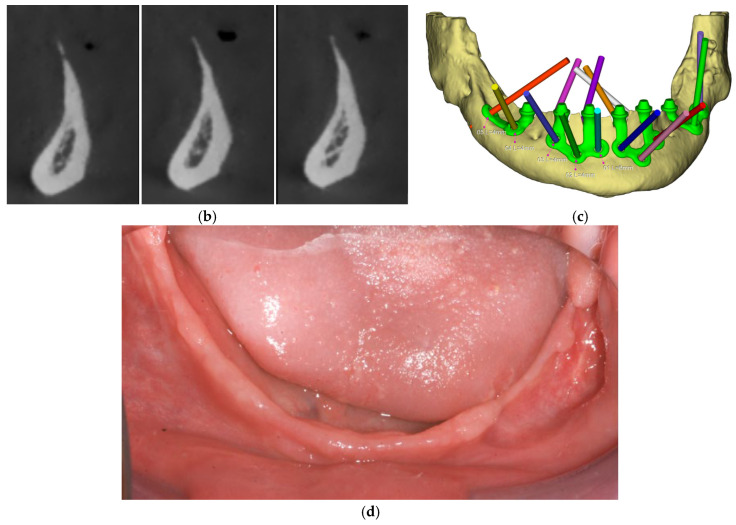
(a) A panoramic radiograph of the patient at the initial stage was obtained to evaluate the overall dentition, bone structure, and any developmental anomalies. (b) Cross-sectional images show a severely resorbed, knife-edged alveolar bone crest. (c) Digital planning of the subperiosteal implant design and screw positioning. (d) Intraoral clinical image showing a completely edentulous knife-edged alveolar ridge.
